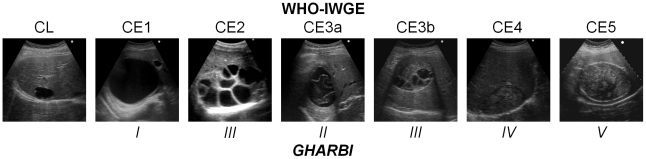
Comparison of Gharbi's and WHO-IWGE ultrasound classifications of CE cysts. CL, as a potentially parasitic cyst, was not in Gharbi's classification and needs to be differentiated from non-parasitic cysts. This may also happen with CE1 cysts, when the double layer sign is not evident. Also, WHO CE3b had not been explicitly described by Gharbi but could likely be classified as Type III.
